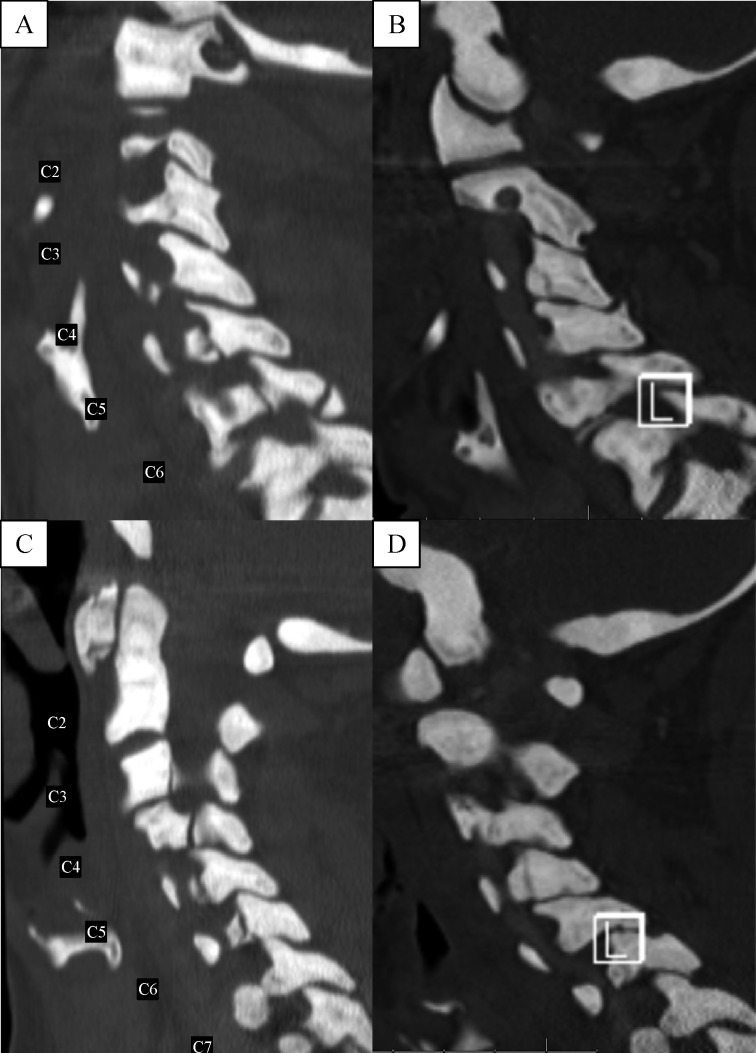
CT cervical spine sagittal sequences.
